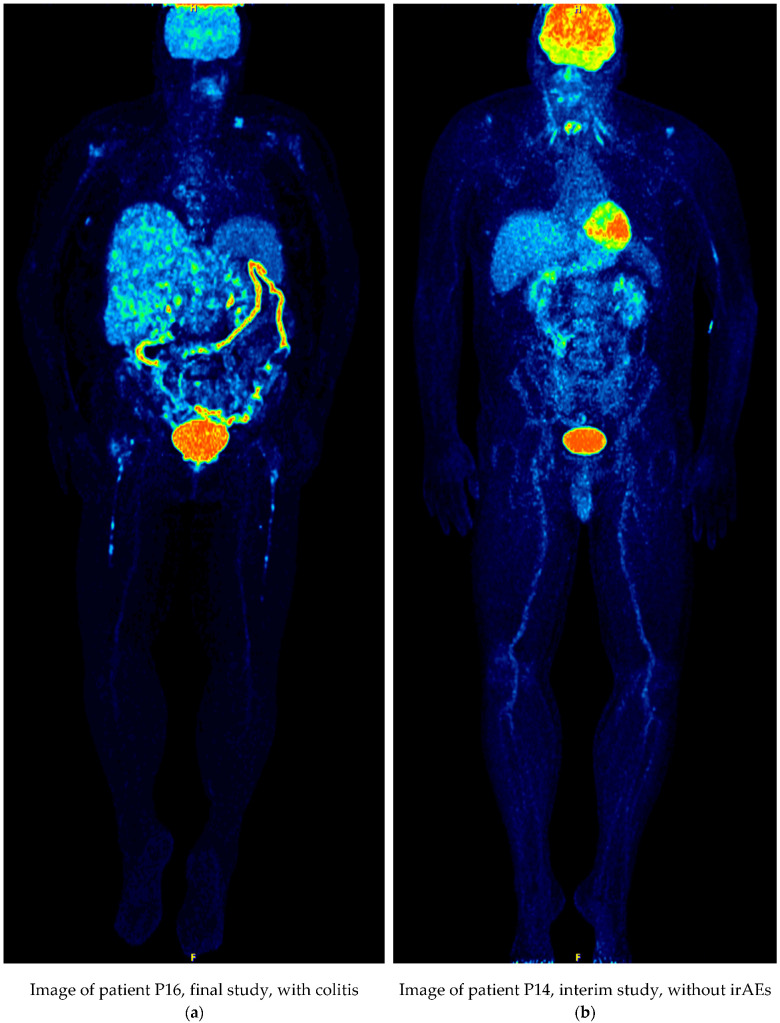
MIP PET-CT images for patients with metastatic melanoma: (a) Patient P16 at final stage with signs of colitis; (b) Patient P14 at interim stage without irAEs effects.
